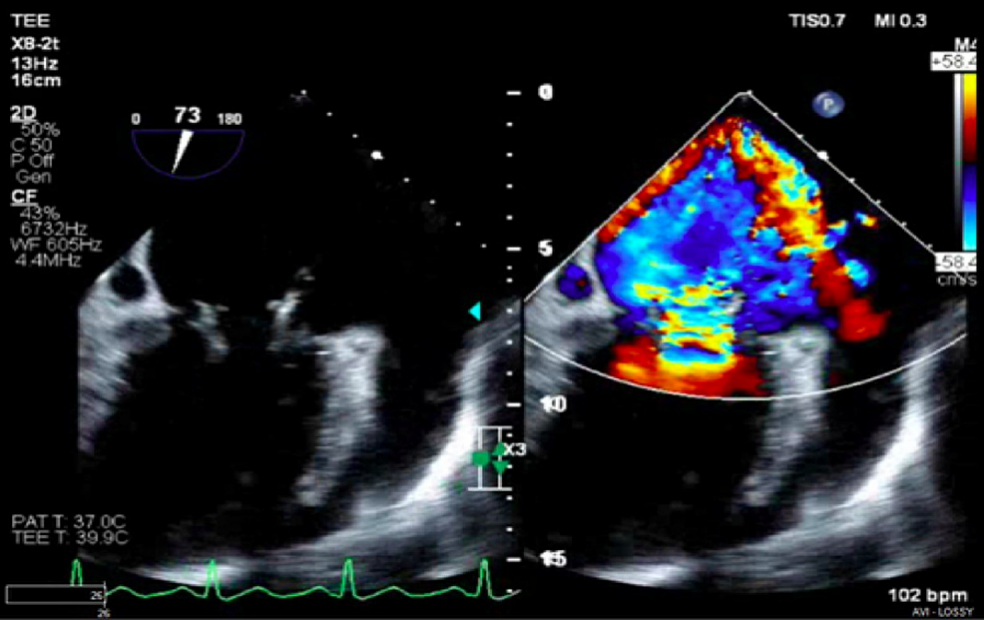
2.1 cm mitral valve leaflet mobile vegetation viewed on transesophageal echocardiogram (TEE)
